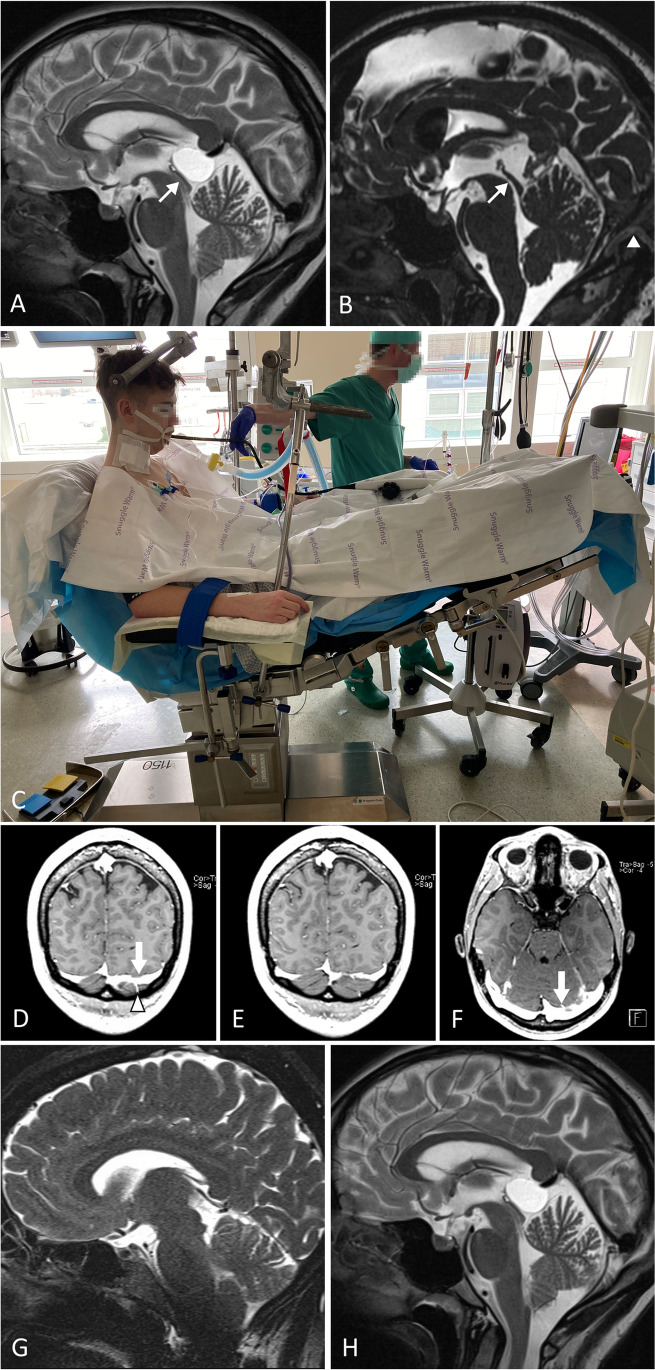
Midsagittal MR CISS before (A) and after surgery (B) demonstrating a pineal cyst leading to a narrowed Sylvian aqueduct (arrow) without hydrocephalus. Patient complaining of longstanding and increasing headache attacks. Post-operative MR CISS (B) midsagittal image: complete cyst resection; open Sylvian aqueduct. Craniotomy reaching the transverse sinus (arrow-head). Symptoms resolved completely. C Semi-sitting position. Contrast-enhanced T1-weighted MR (coronal (D, E), axial (F)): left-sided predominant sinus and bridging vein; decision for approach on right side with the higher-rising sinus. Variations of steepness of tent: paramedian (G) viewing trajectory to the pineal region is not as steep as in the midline (H) approach
